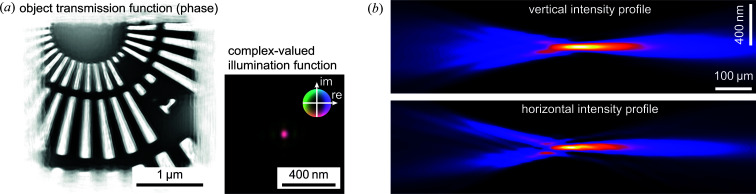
Characterization of a nanofocused X-ray beam by ptychography using the previous nanoprobe setup on beamline P06. (*a*) The illumination and object function were reconstructed using the ePIE algorithm. (*b*) Caustics of the nanofocused X-ray beam in the vertical and horizontal planes.
